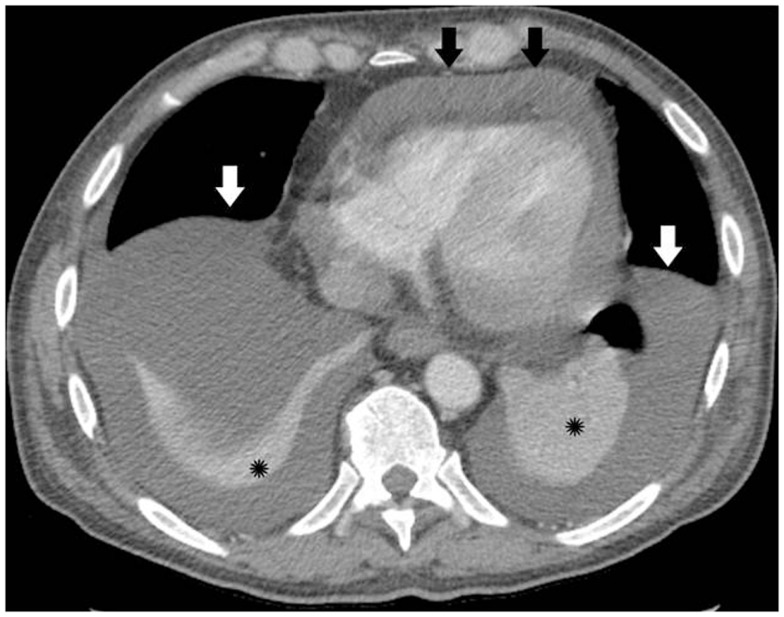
Pre-treatment computed tomography (CT) image with intravenous (IV) contrast, demonstrating bilateral pleural effusions (white arrows) and moderate-sized pericardial effusion (black arrows), prior to thoracentesis, pericardiocentesis, or chemotherapy. Black asterisk = atelectatic lung.
